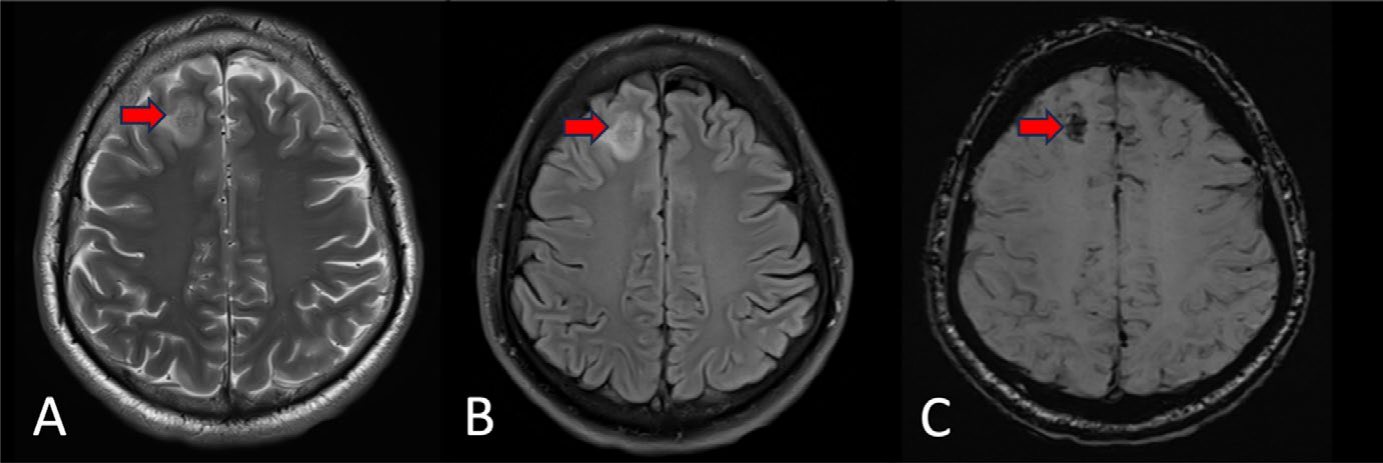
(A) T2‐TSE‐AXIAL MRI and (B) T2‐TSE‐FLAIR (dark fluid) MRI showed right high frontal cortical/juxta‐cortical lesion with mixed signal intensity and surrounding vasogenic edema, (C) SWI‐axial MRI showed extensive abnormal blooming (the red arrows) denoting for hemorrhagic component.
